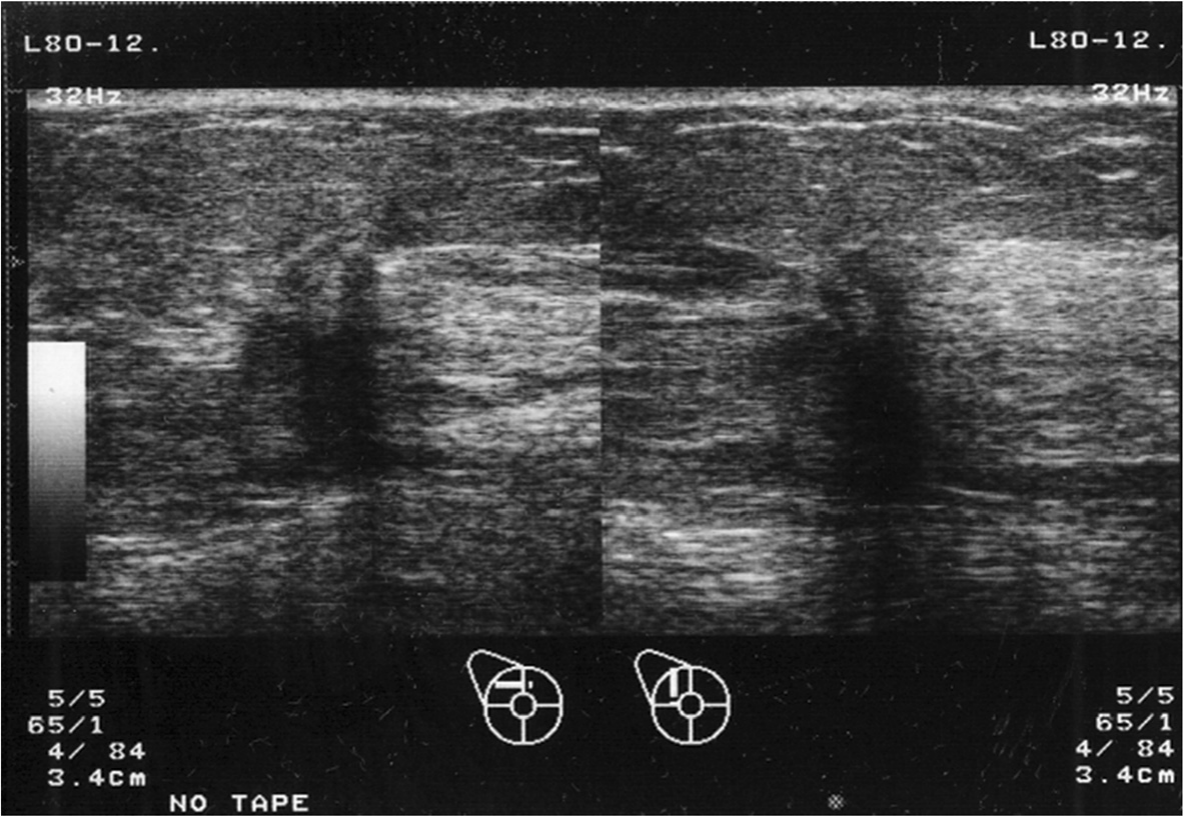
A ductal ultrasonogram showing an irregularly shaped mass of approximately 5 × 6mm with unclear boundaries in area C of our patient’s right breast. The internal echogenicity was uneven and the posterior echo was dissipated.
